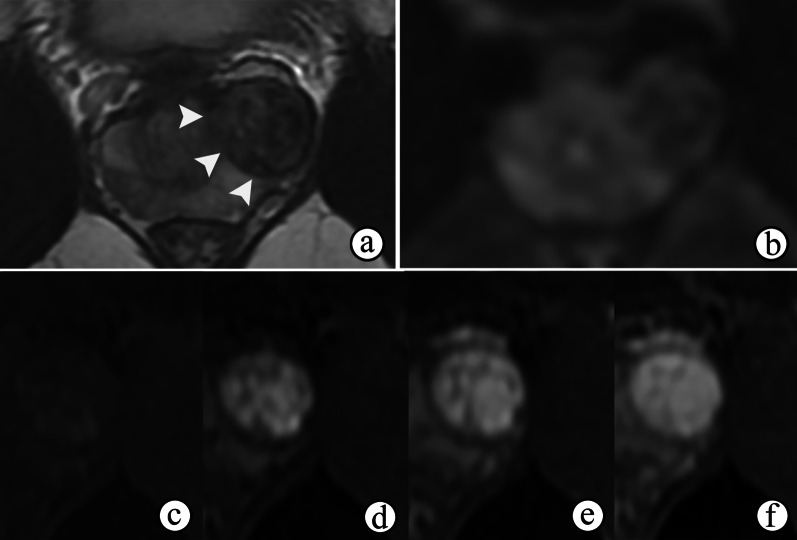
Prostate stromal tumor of uncertain malignant potential in a 39-year-old man with a normal serum PSA level of 1.780 ng/mL. a Axial T2WI shows a well-circumscribed nodule with heterogeneous signal and an incomplete capsule (arrowheads) located in the left PZ of the prostate and compressing the extra-prostatic tissue. b DWI shows hypointensity, indicating no restricted diffusion of the nodule. c–f Axial DCE imaging at 30 s (c), 60 s (d), 120 s (e) and 180 s (f) after contrast injection shows the early and gradual enhancement of the nodule
